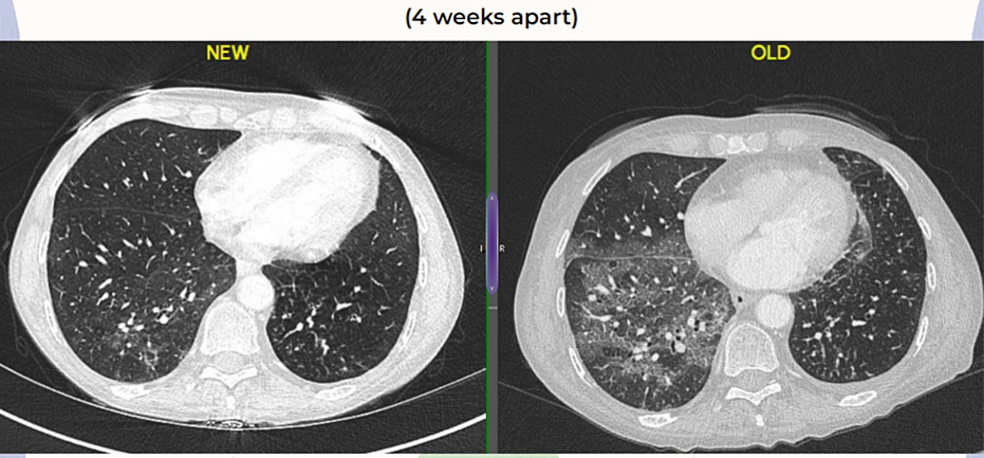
Comparative CT chests four weeks apart
